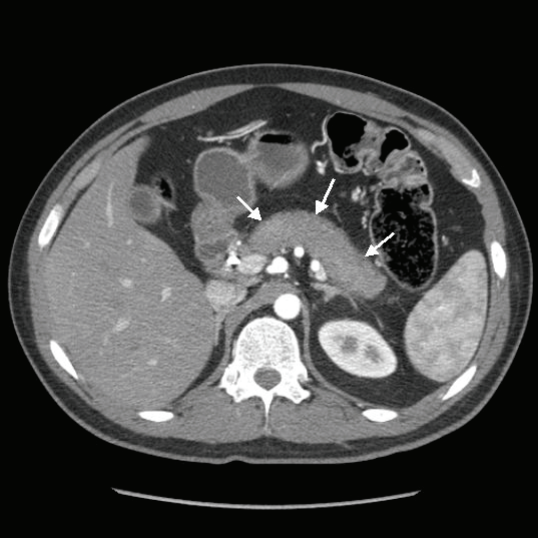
Computed tomography of the abdomen showing homogeneous parenchymal enhancement with an almost "feathery" border and "sausage" appearance of the pancreas.
